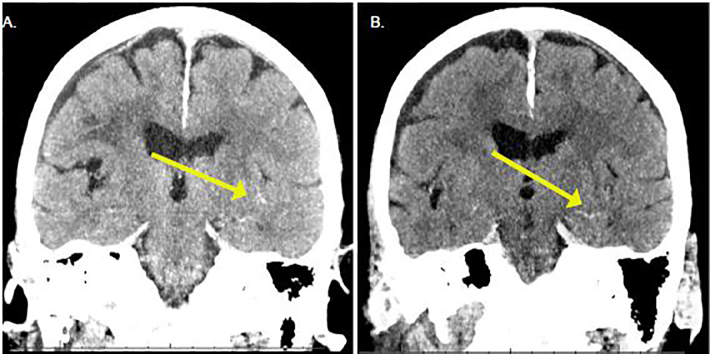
Coronal computed tomography demonstrating trace blood (arrows) at presentation (A) and four hours later (B) showing no progression of bleeding.
